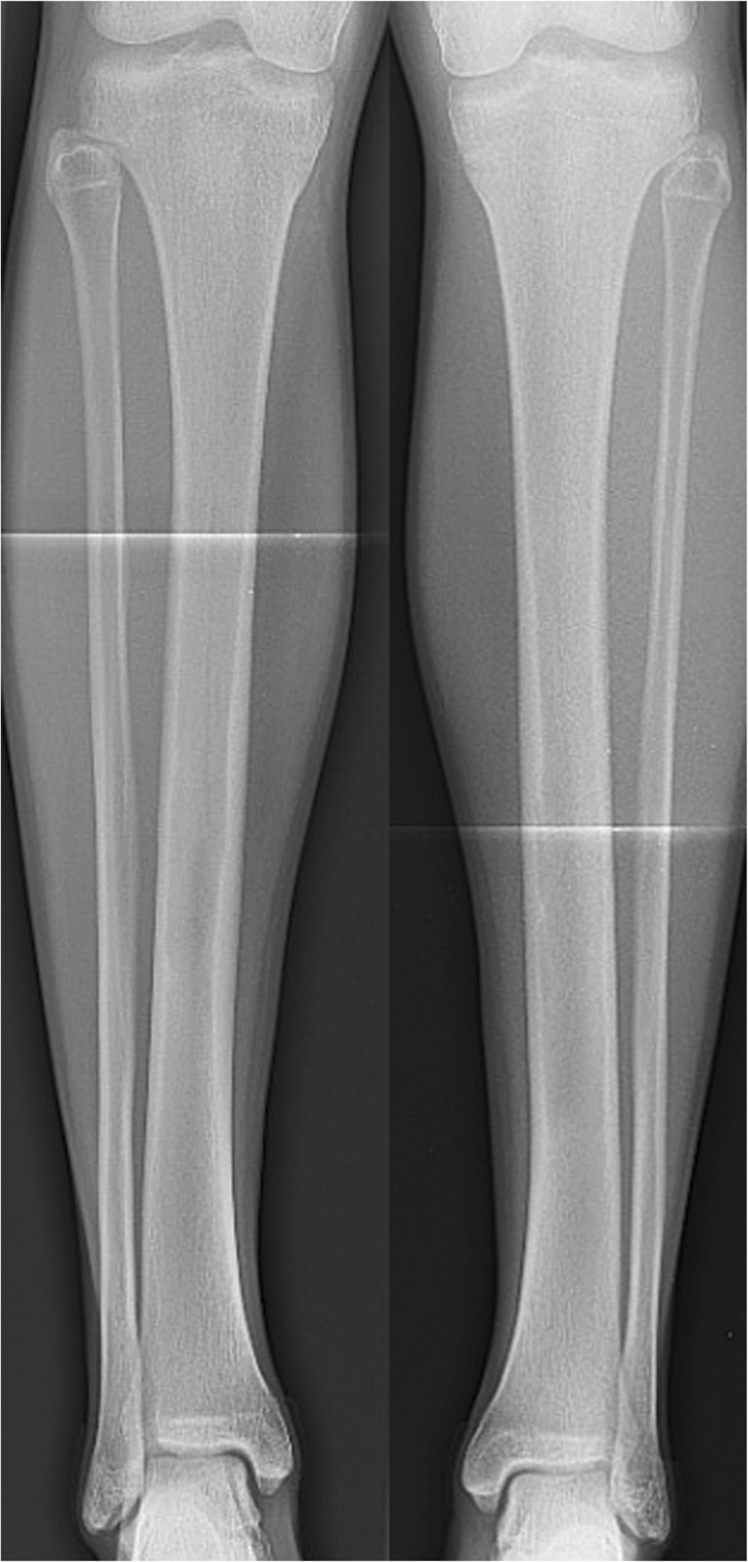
Plain radiography (a.p.) of both tibiae. Here, tender signs of reduced radiopacity with irregular cortical involvement and an expansive character of the lesion could be observed in both distal diaphyses
